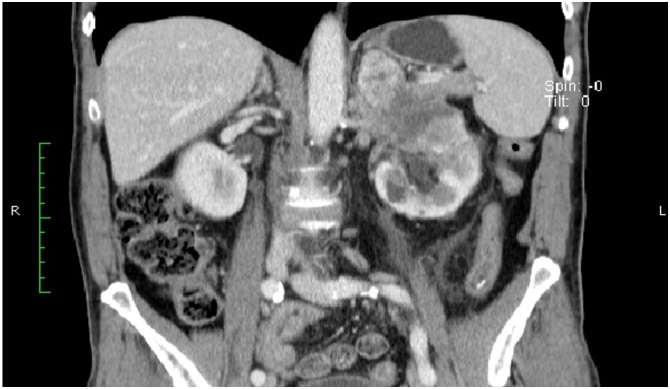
Abdominal CT revealed an 8 cm left renal growth suggestive of neoplasia.
